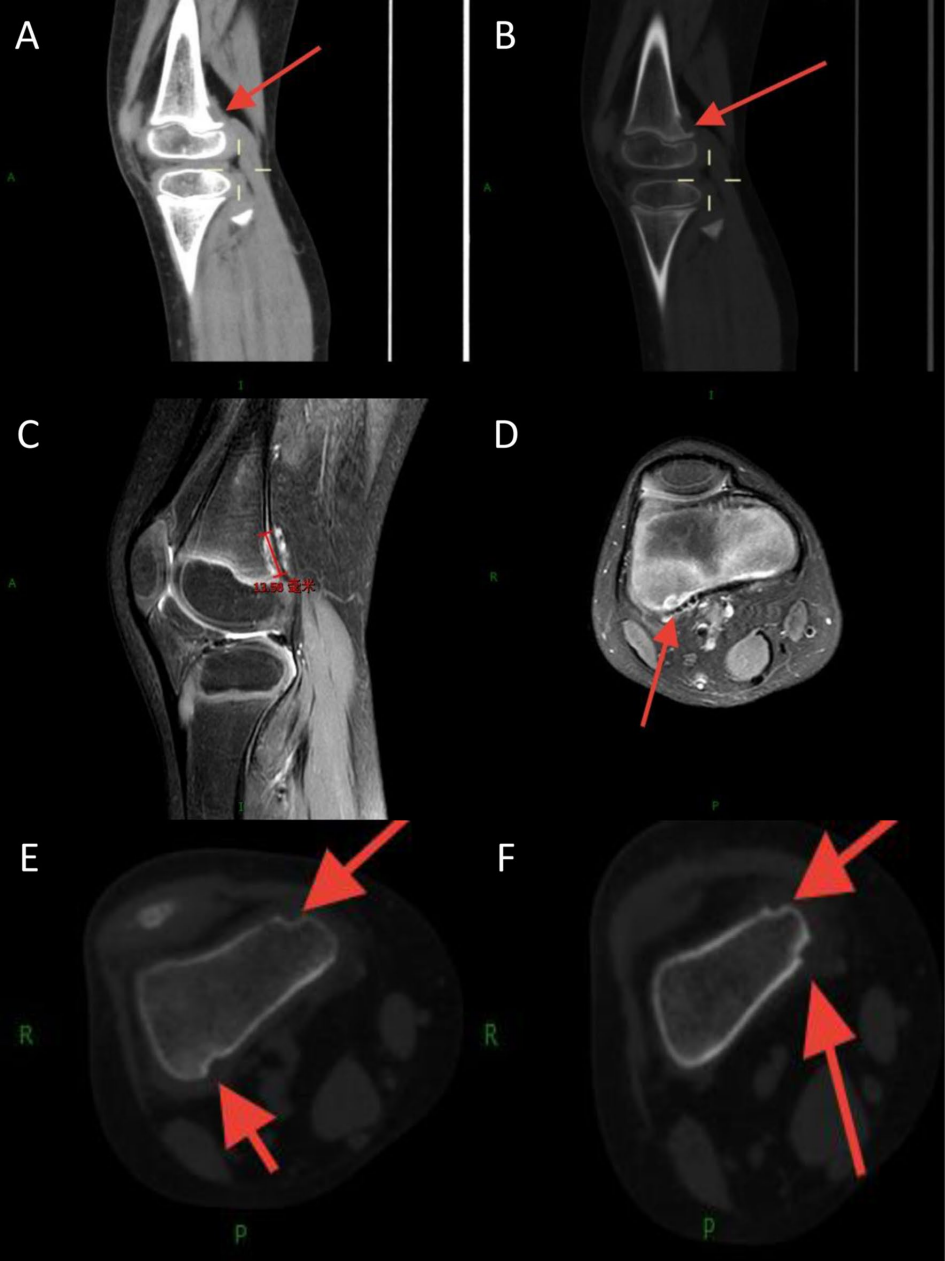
Cortical excavation pattern (Pattern 2) of KHE in a 7-year-old male with chronic knee pain. (A) Axial CT image (bone window) of the distal femur shows a focal, saucer-like excavation of the posteromedial cortex (white arrow), with an irregular base. (B) Sagittal CT reconstruction better delineates the “apple bite” appearance of the cortical defect (black arrow). (C, D) Sagittal and axial proton density-weighted fat-suppressed (PD-FS) MRI sequences show high signal intensity at the site of the cortical erosion (white arrows), with minimal adjacent marrow edema and no associated soft-tissue mass
